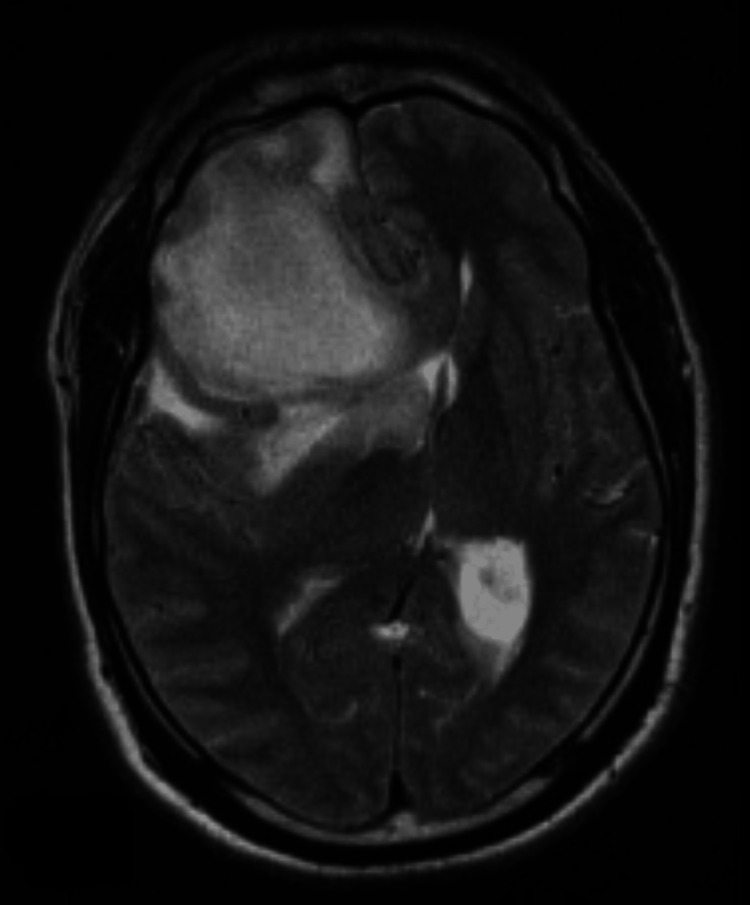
T2-weighted images demonstrating hyperintense mass. There is moderate vasogenic edema in the right frontal lobe and severe mass effect with right-to-left midline shift.
